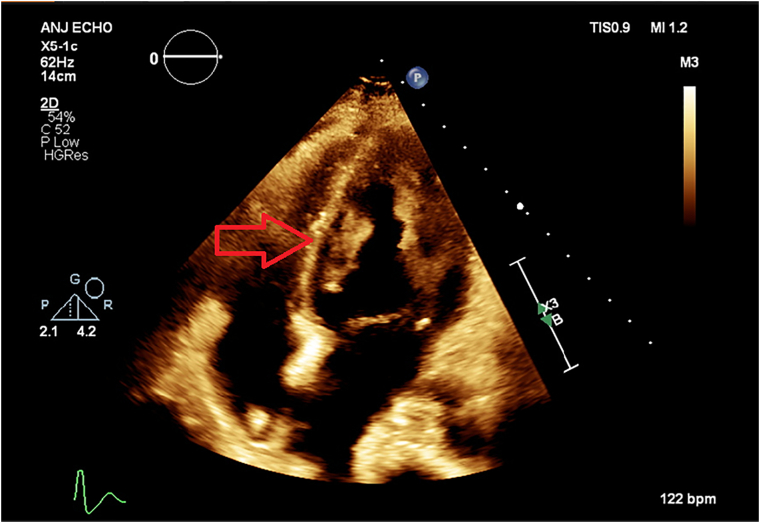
Three-chamber transthoracic echocardiography showing a mobile echogenic structure at the inferolateral wall of the left ventricle extending to the mitral valve.
